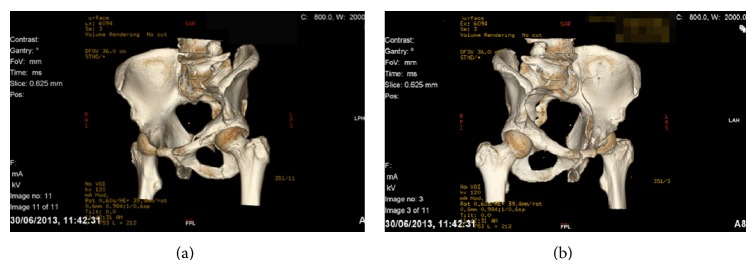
(a) An obturator oblique CT reconstruction. (b) An iliac oblique CT reconstruction.
